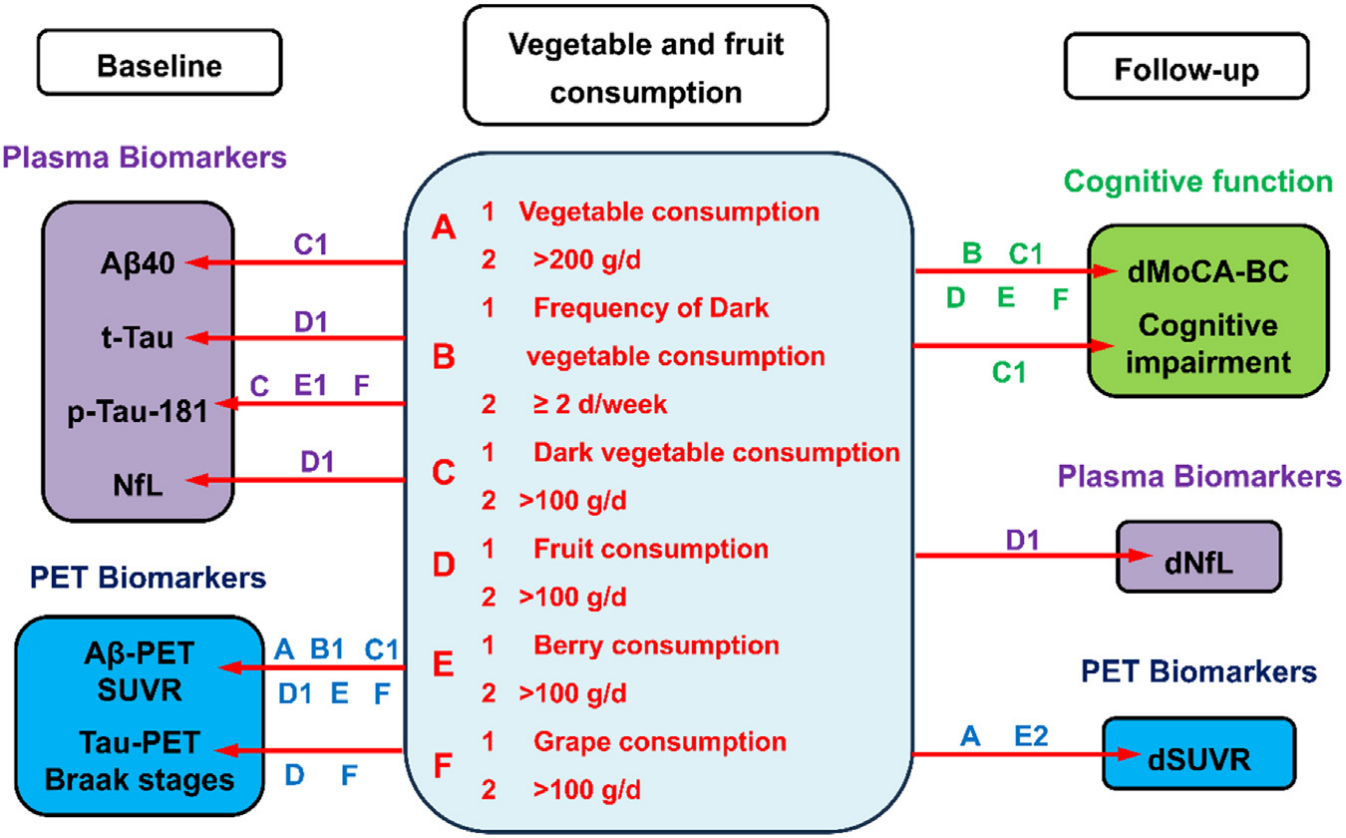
The associations between vegetable and fruit consumption and the biomarkers in the cross-sectional study as well as the changes of cognitive function and biomarkers in the longitudinal study of the cognitively unimpaired participants with Aβ-PET (+). If the associations were simultaneously observed in both “1″ and “2″ of each section, only the capital letters (A-F) are labeled. “d”, difference between the follow-up and baseline data; MoCA-BC, Chinese version of Montreal Cognitive Assessment-Basic; NfL, neurofilament light chain; PET, positron emission tomography; SUVR, standard uptake value ratio.
